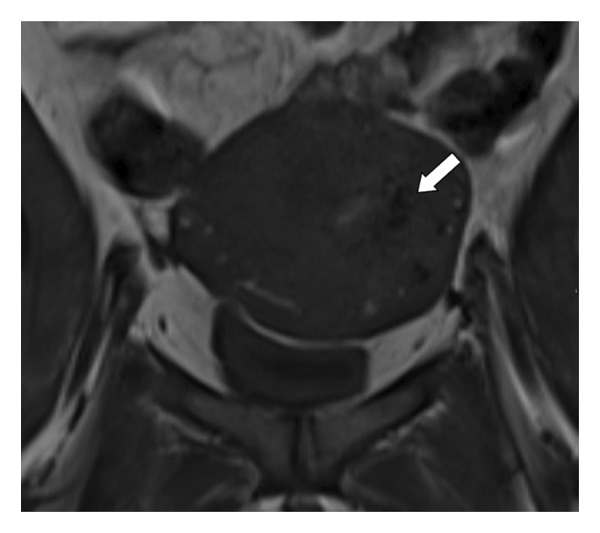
Coronal MRI of the pelvis (T1‐weighted) shows multiple serpentine flow signal voids in the left uterine wall, endometrial cavity, and parametrium features concerning for uterine arteriovenous malformation.
